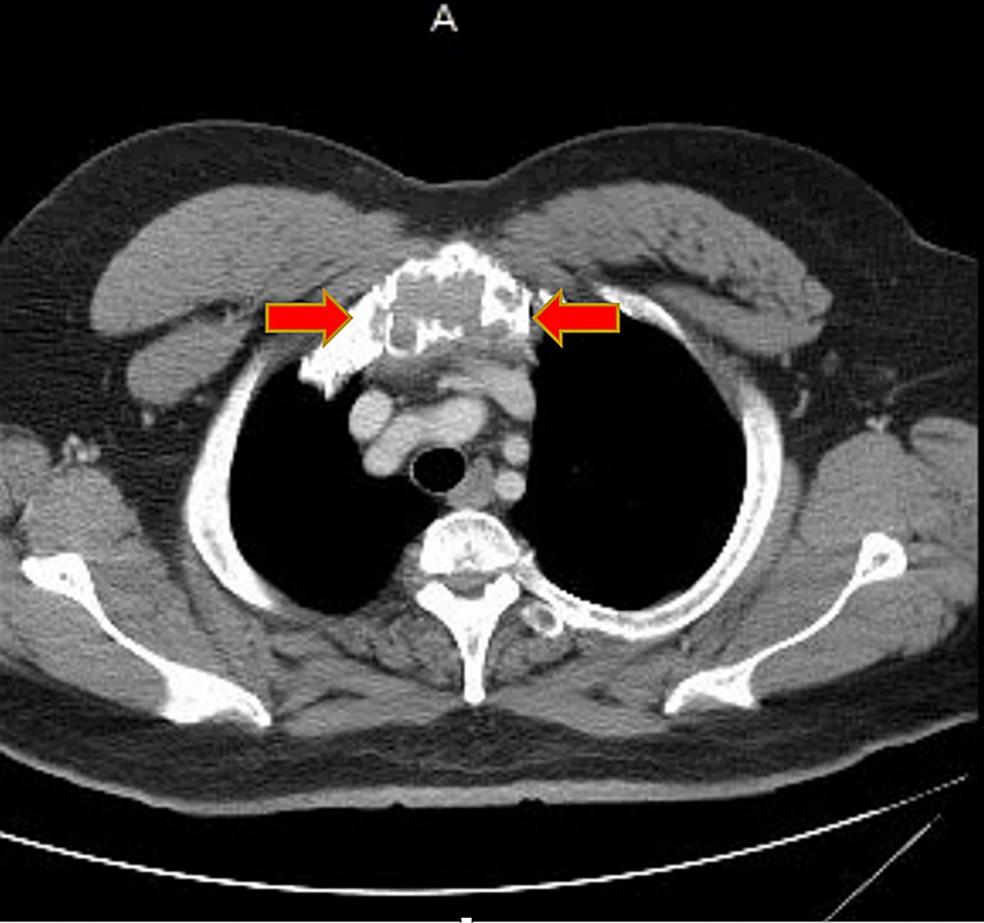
CT scan showing expansile, lytic lesion involving the manubrium (red arrows) A - anterior
